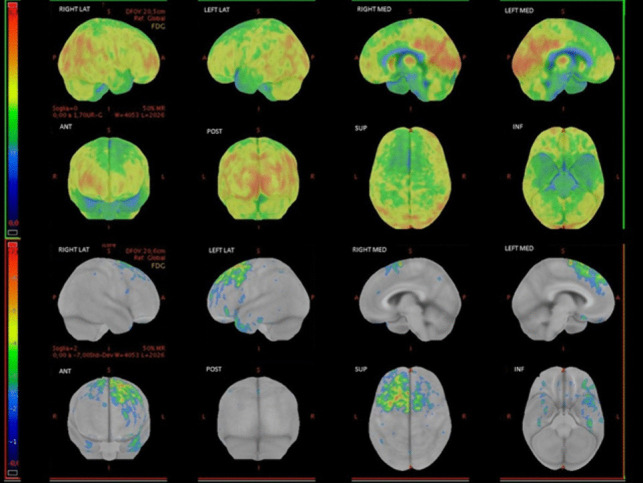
FDG-PET of subject II-3, showing left fronto-temporal hypometabolism. Images have been obtained with CortexID suite (GE Healthcare). Hypometabolic areas appear in cold tones in the upper rows, representing FDG uptake. The lower rows represent the z-score map obtained by comparison with the software database of normal subjects: the higher the z-score (red), the more pronounced the reduction of the metabolism
